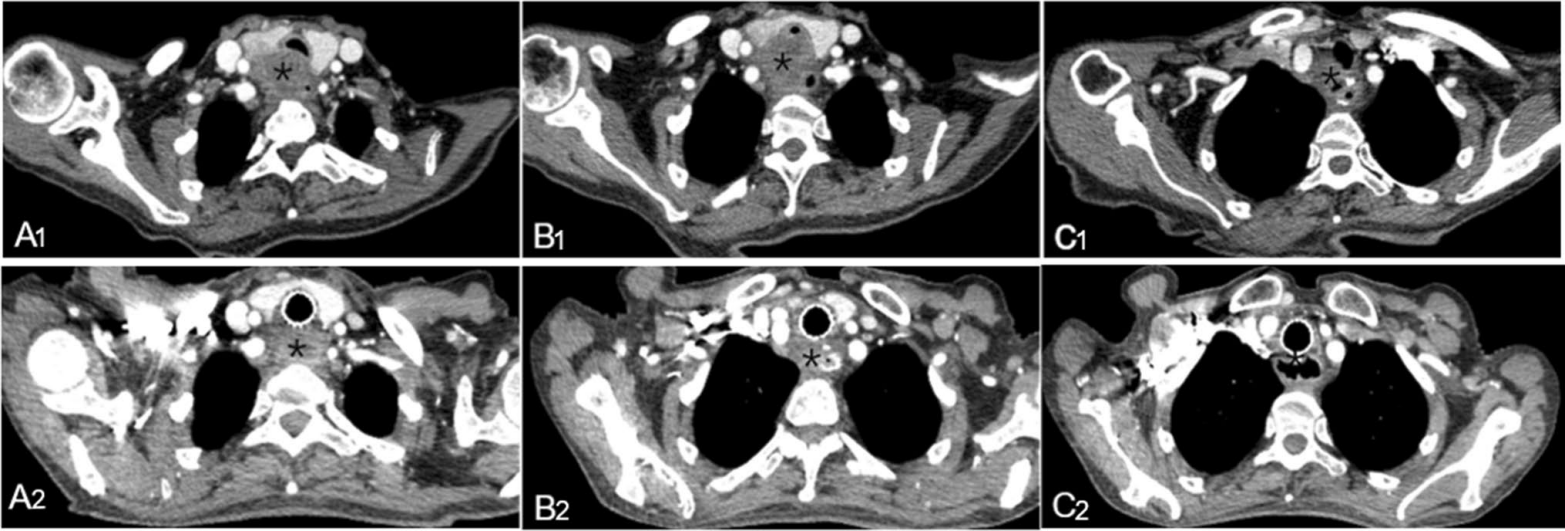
A 65-year-old female presented with dyspnea for more than one week after palliative resection of esophageal cancer. Before airway stent implantation, CT (A1, B1, C1) showed a soft tissue mass on the right side of the anastomosis with an irregular edge and moderate heterogeneous enhancement, and the trachea was compressed and narrowed forward. One month after TAIC, CT (A2, B2, C2) showed a soft tissue mass on the right side of the anastomosis, with moderate uneven enhancement, which was significantly smaller than before, and the stent shadow can be seen in the trachea. The asterisks in the figures represent the location of tumor invasion.
